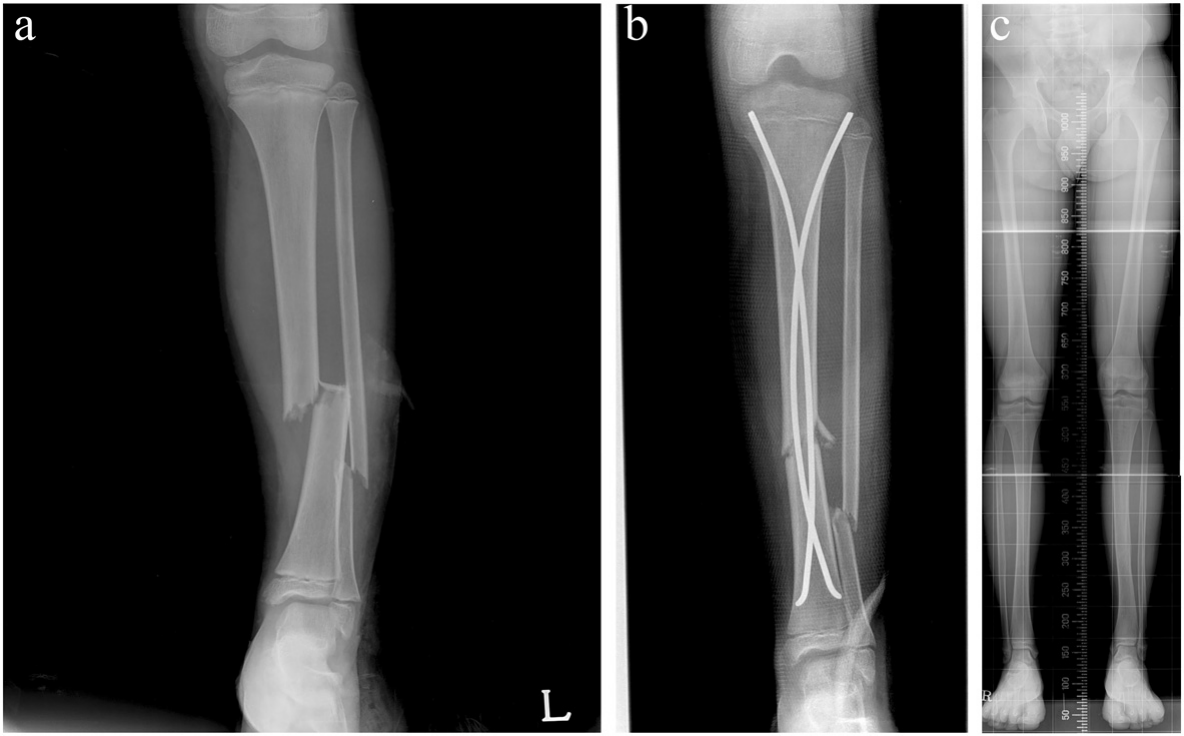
Serials radiographs of an eight-year-old boy who sustained a left distal tibiofibular fracture. (a) Anteroposterior radiograph at trauma. (b) Immediate postoperative radiograph. Treatment involved flexible intramedullary nailing. Postoperative angulation was 8° valgus. (c) A standing orthoroetgenogram after 5 years shows persistent valgus angulation.
